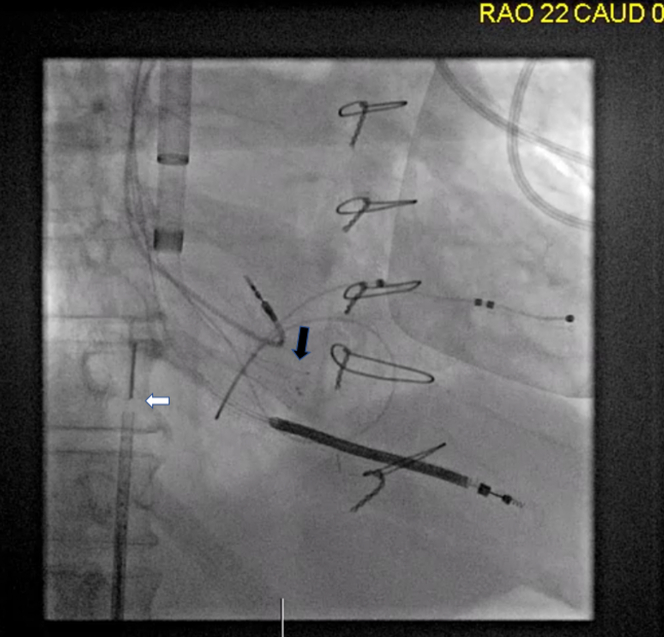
Fluoroscopic Image Demonstrating Catheter-Based Thrombus/Vegetation Aspiration The black arrow highlights the AlphaVac aspiration device positioned within the right atrium, and the white arrow indicates the intracardiac echocardiography catheter.
